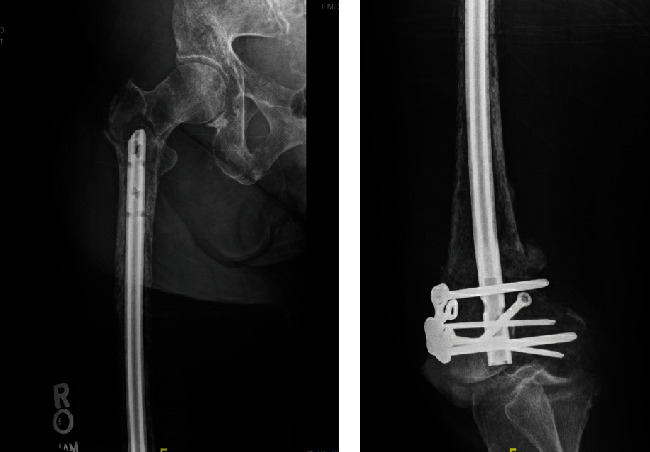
Radiograph of femur revealing right pathologic peri-implant intertrochanteric femur fracture.
